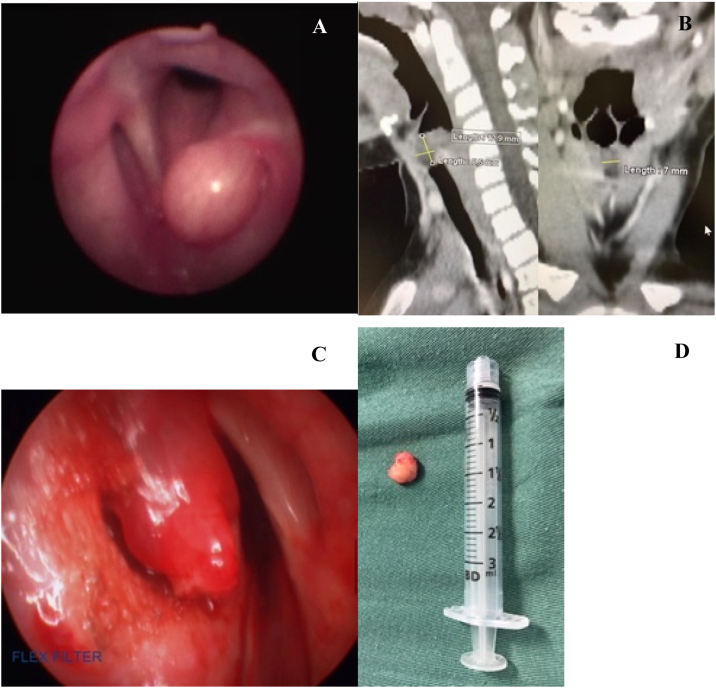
(A) Flexible fiber-optic laryngoscopy revealing submucosal swelling in the left ventricle. (B) Computed Tomographic (CT) scan of the neck revealing a submucosal hypodense supraglottic mass on the left side of the airway, measuring 8.5 × 12.9 × 7 mm. (C) View of the rigid laryngoscopy after removal of the submucosal mass with the preservation of the mucosal flap and (D) macroscopic appearance of the excised lesion.
